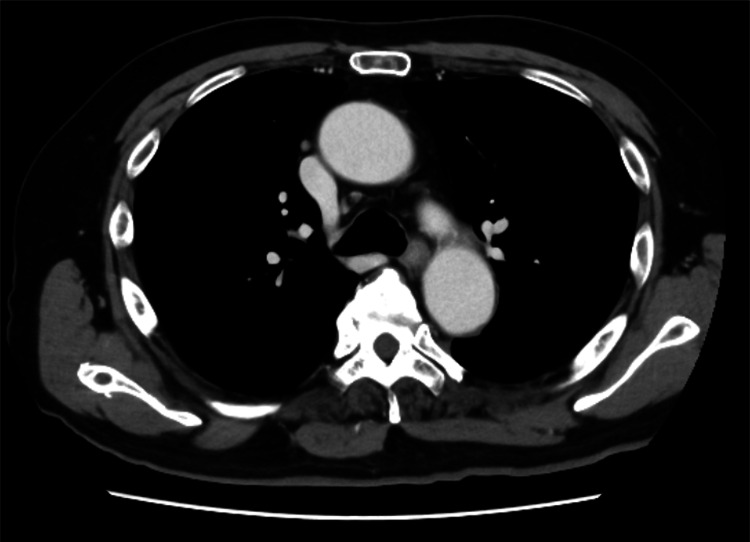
Contrast-enhanced CT of the chest. Contrast-enhanced CT of the chest revealed no abnormalities, including mediastinal masses suggestive of thymoma.
